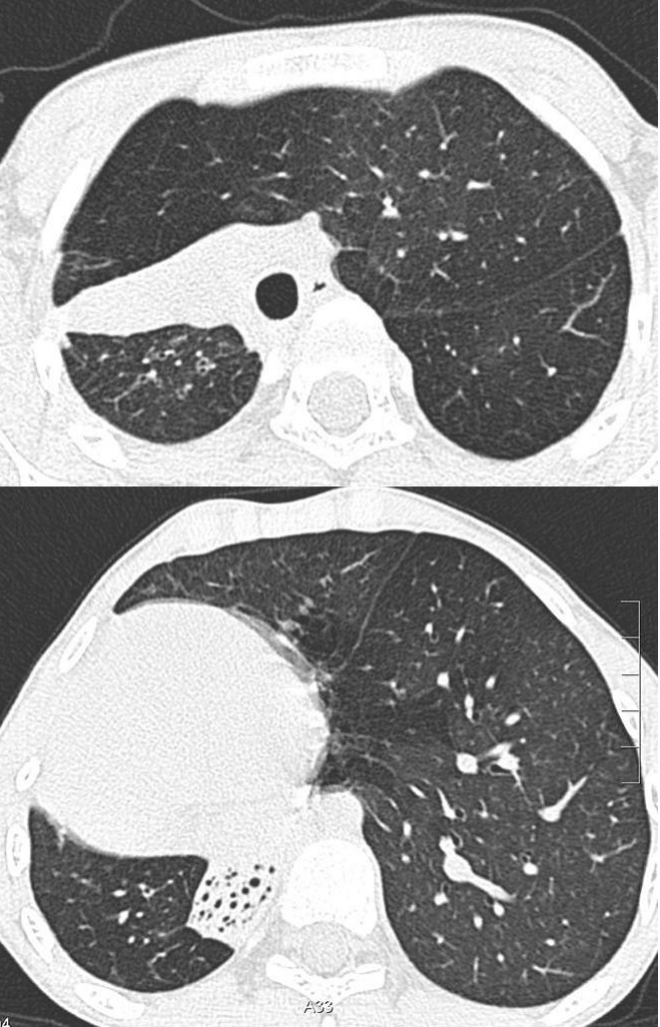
A High resolution computed tomography demonstrates partial re-expansion of the right lung with very moderate bronchiectasis. B The right lower lobe remains collapsed and shows more extensive bronchiectasis.
